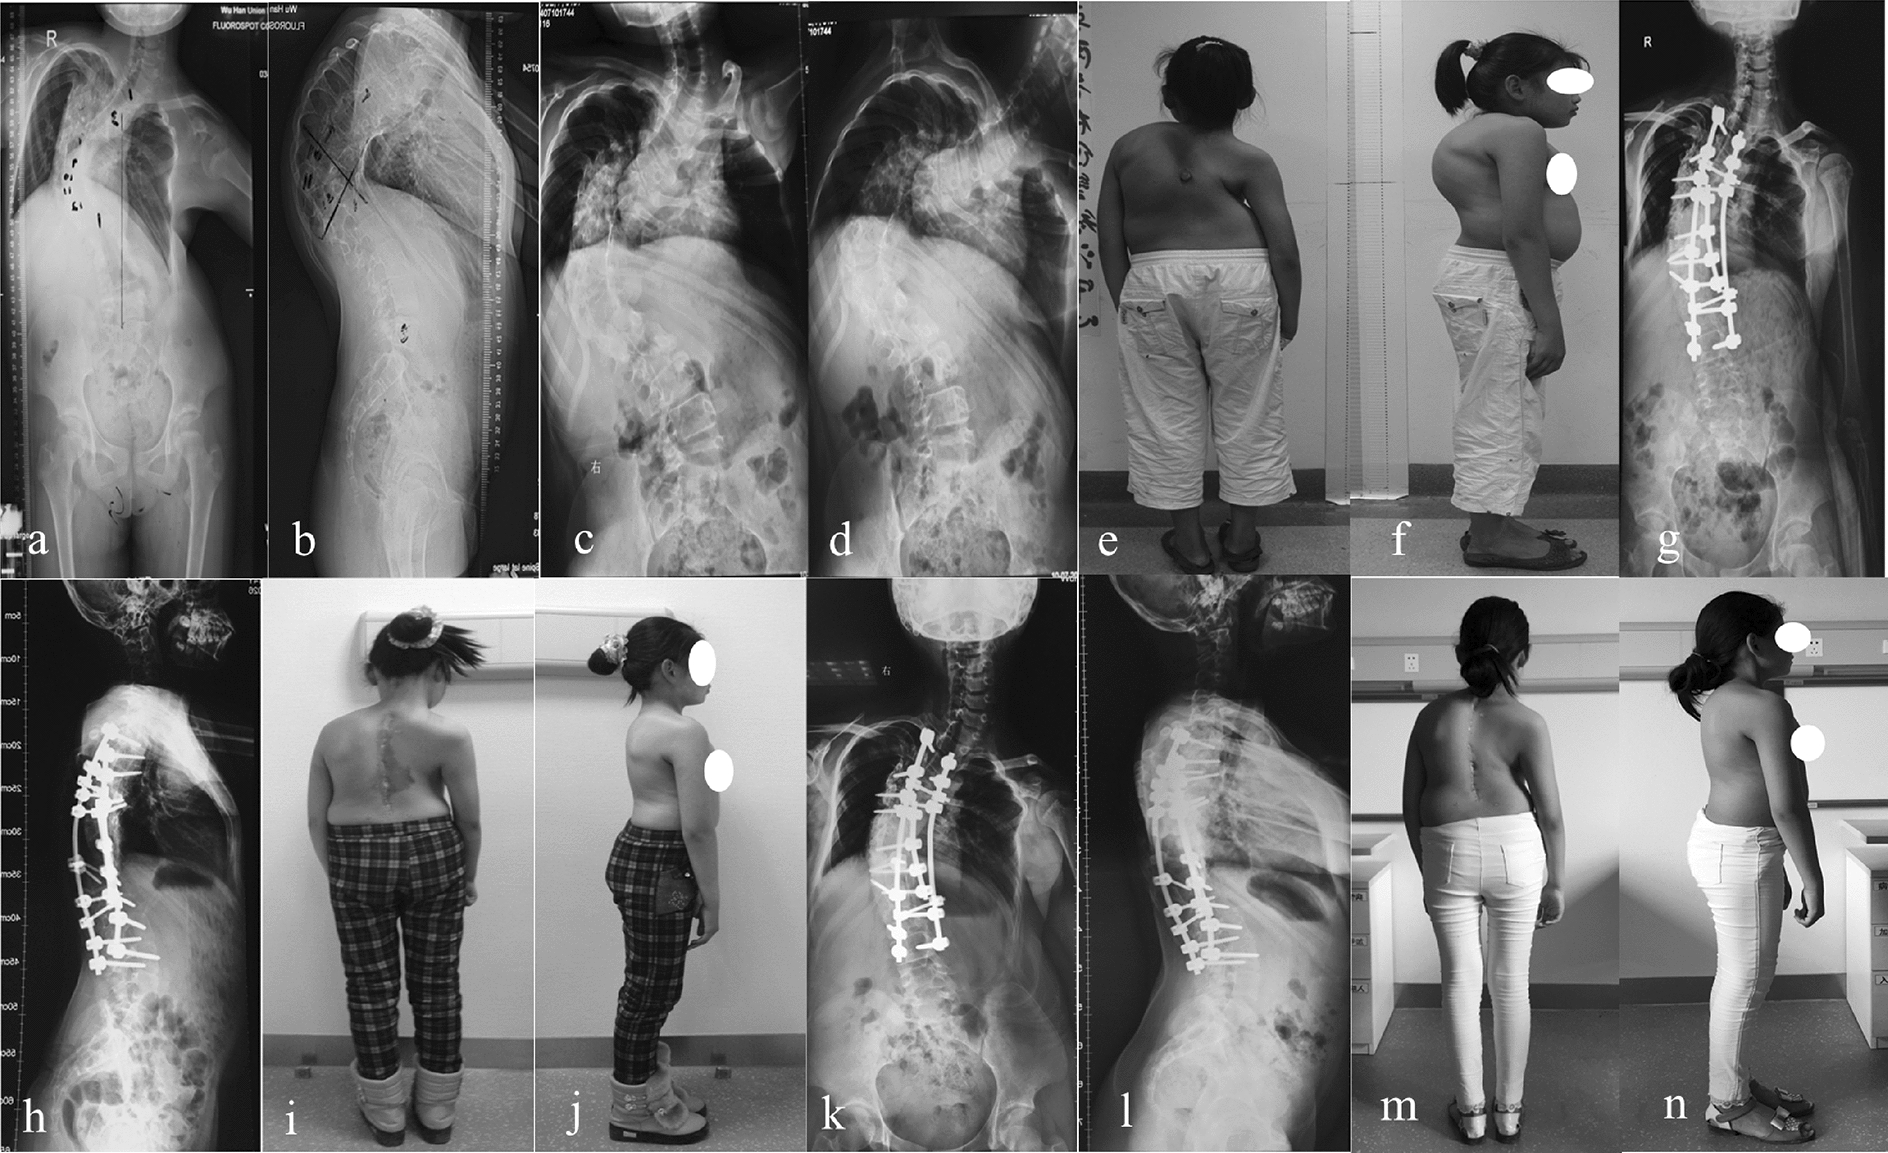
Standing radiographs and images of a 13-year-old female with severe rigid spinal deformity. a–d Preoperative radiographs; e–f preoperative appearance in posterior and lateral view; g–h postoperative anterior–posterior and lateral radiographs; i–j postoperative appearance in posterior and lateral view; k–l anterior–posterior and lateral radiographs at the final follow-up; m–n posterior and lateral appearance at the final follow-up
